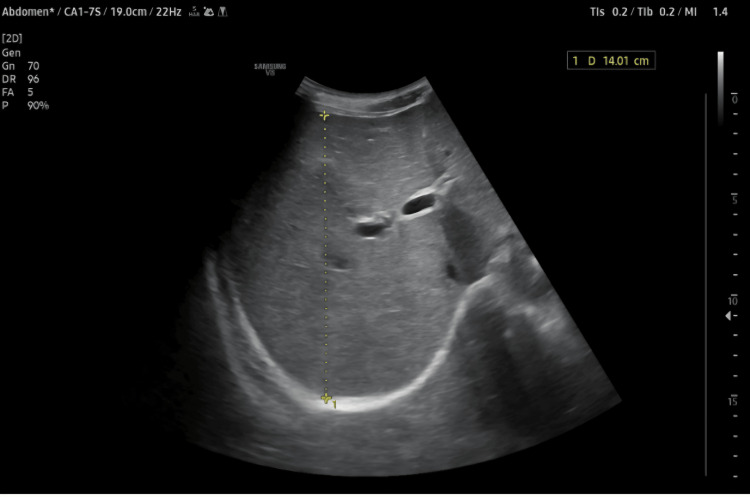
Mild hepatomegaly and prominent hepatic veins on abdominal ultrasound
